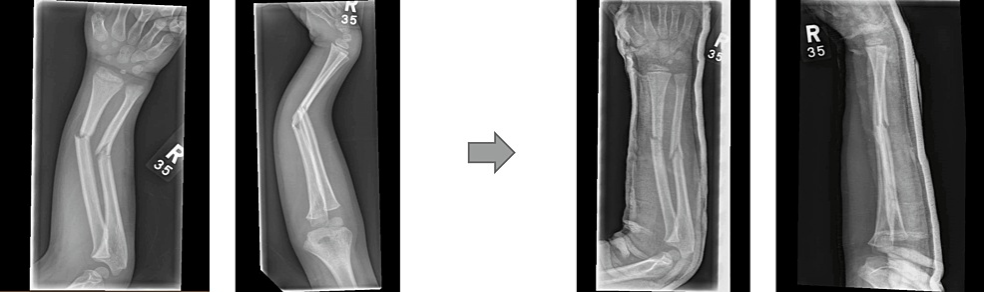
Radiograph 2 showing distal radius/ulna fracture in AP/Lat views and the subsequent manipulated views AP: Anteroposterior; Lat: Lateral
